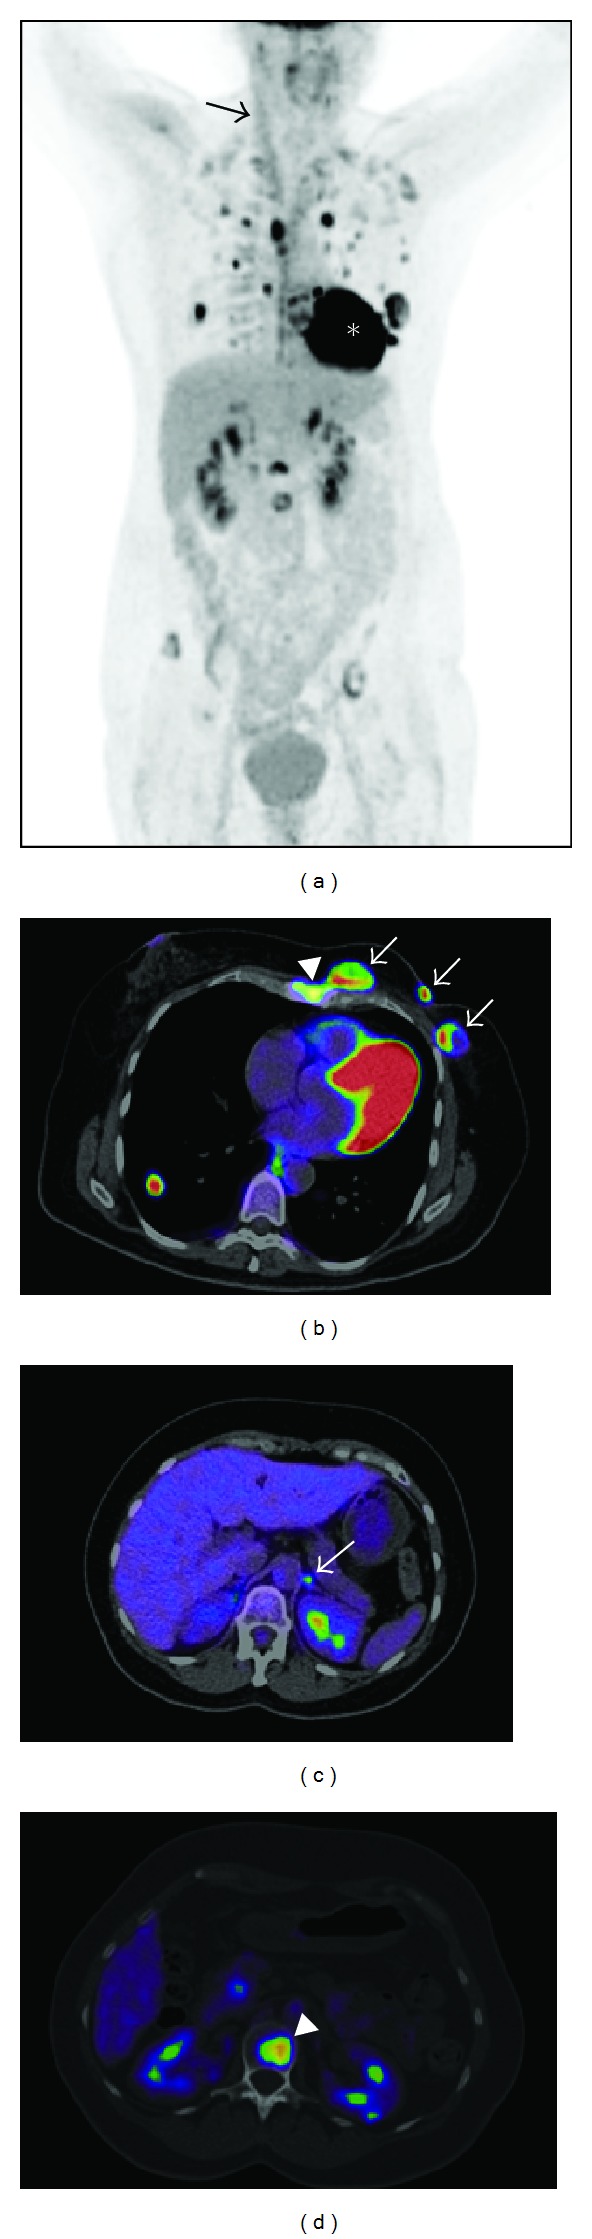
A 47-year-old woman with a left-sided multifocal infiltrating ductal carcinoma, Luminal B, referred for staging. Images were obtained in a Biograph mCT (Siemens) 60 min after i.v. injection of 363 MBq of FDG. (a) FDG-PET whole-body maximum intensity projection: multifocal left-sided breast tumour, lymph node metastasis in the left axilla, left internal mammary chain and mediastinum, left adrenal gland metastasis, multiple bilateral lung metastases, sternal, spine, and pelvic bone metastases. Physiological uptake was identified in paraspinal and supraclavicular fossa brown fat, right sternocleidomastoid muscle (arrow), myocardium (∗), kidneys and bladder. (b) Axial fused FDG-PET/CT image of the multifocal tumour in the left breast (arrows), sternum (arrowhead), and right lung metastasis. (c) Axial fused FDG-PET/CT image of the left adrenal gland metastasis (arrow). (d) Axial fused FDG-PET/CT image of a vertebral metastasis (arrowhead).
